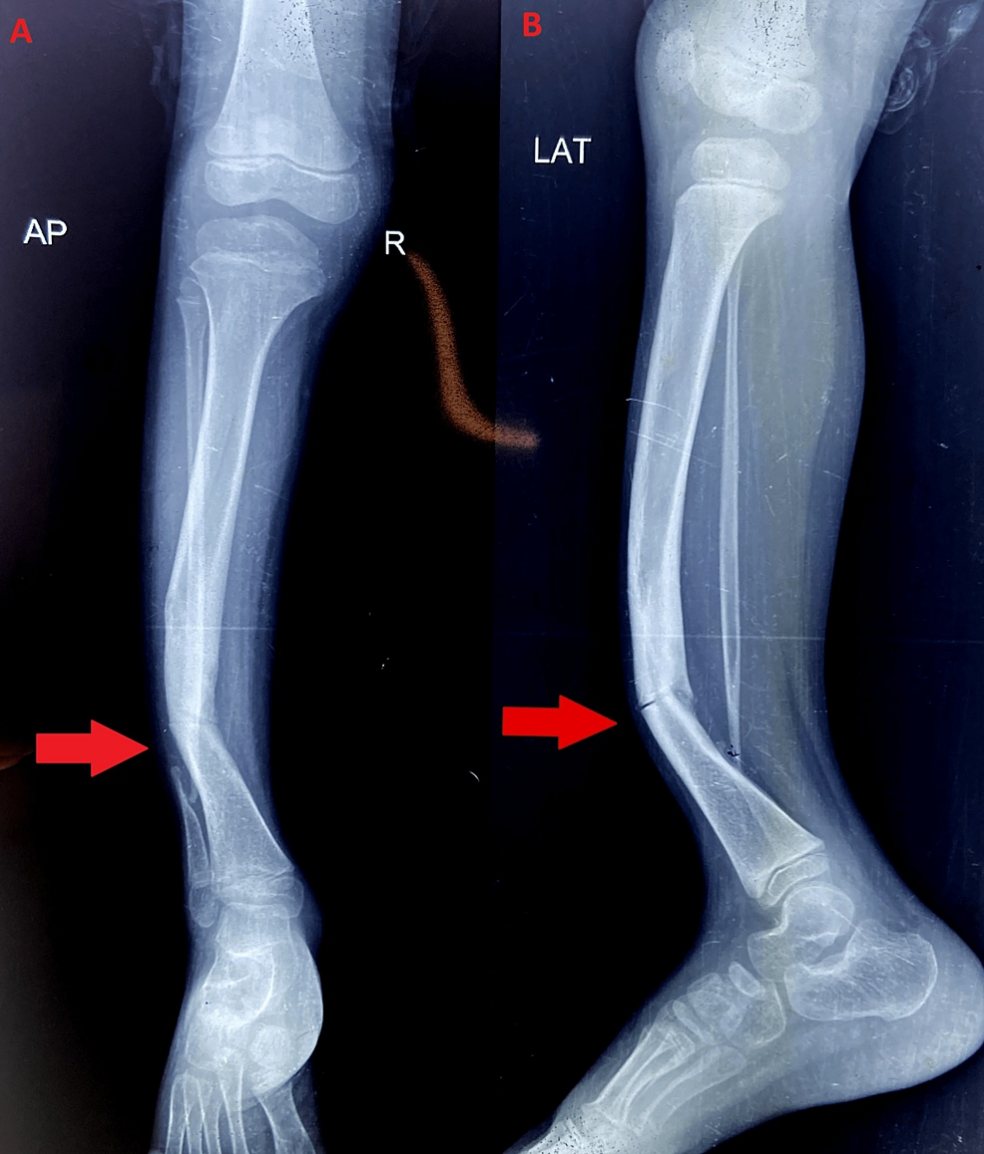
Radiograph showing anterolateral bowing of the tibia. Anterior-posterior and lateral radiographs that were taken before surgery demonstrating anterolateral bowing of the tibia which is the characteristic feature of congenital pseudoarthrosis of the tibia. The author has self-captured.
